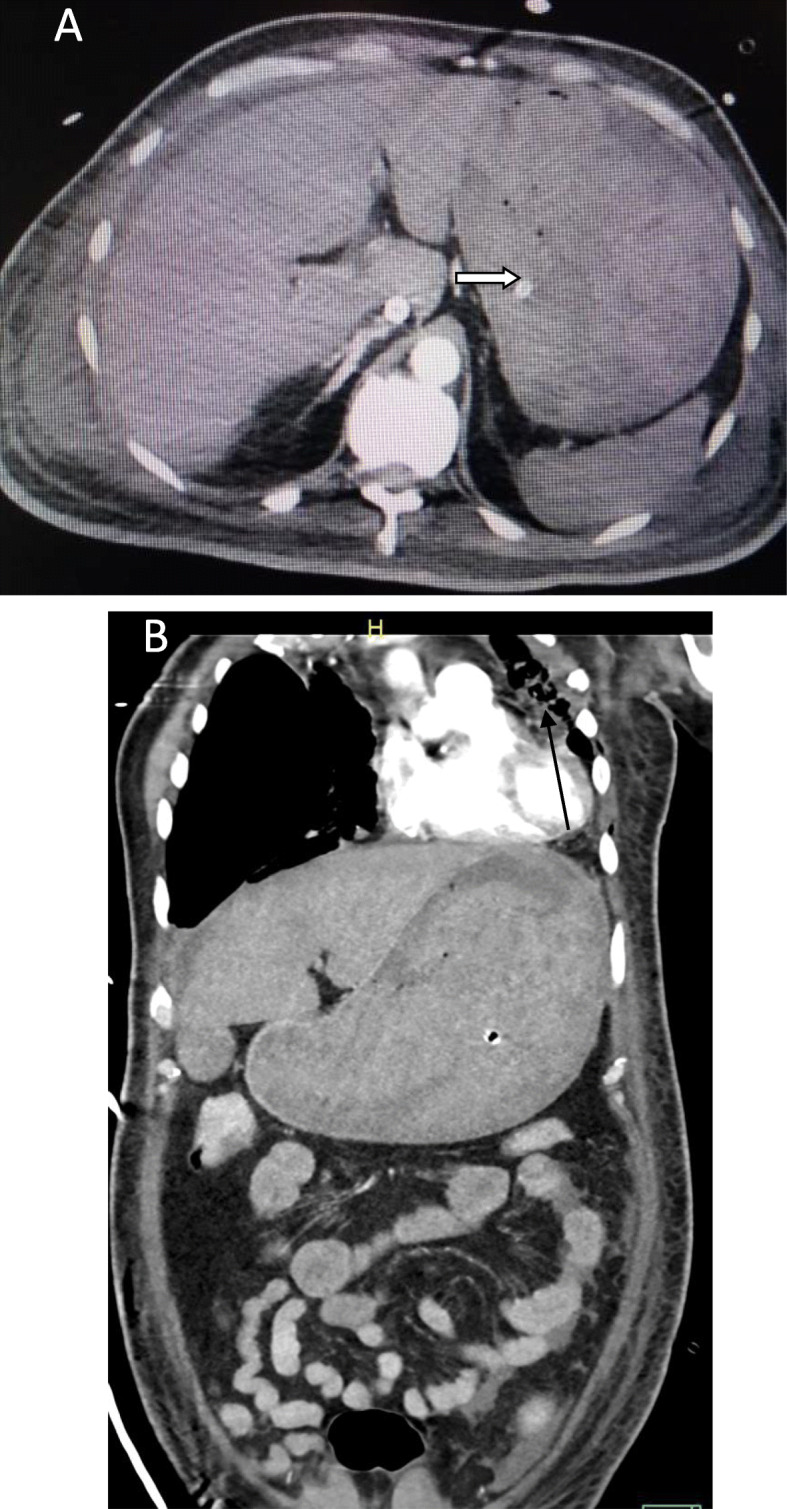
Fifty-six-year-old confirmed COVID-19 male patient admitted to the ICU presented with two attacks of acute hematemesis. a Axial CT of the abdomen and b coronal reconstruction done. The stomach is loaded with blood clots yet no signs of active contrast extravasation in the arterial phase of the study; the nasogastric tube is seen in place (open arrow in a); there are parenchymal lung changes in COVID-19 noted in the left lung field (arrow in b)
